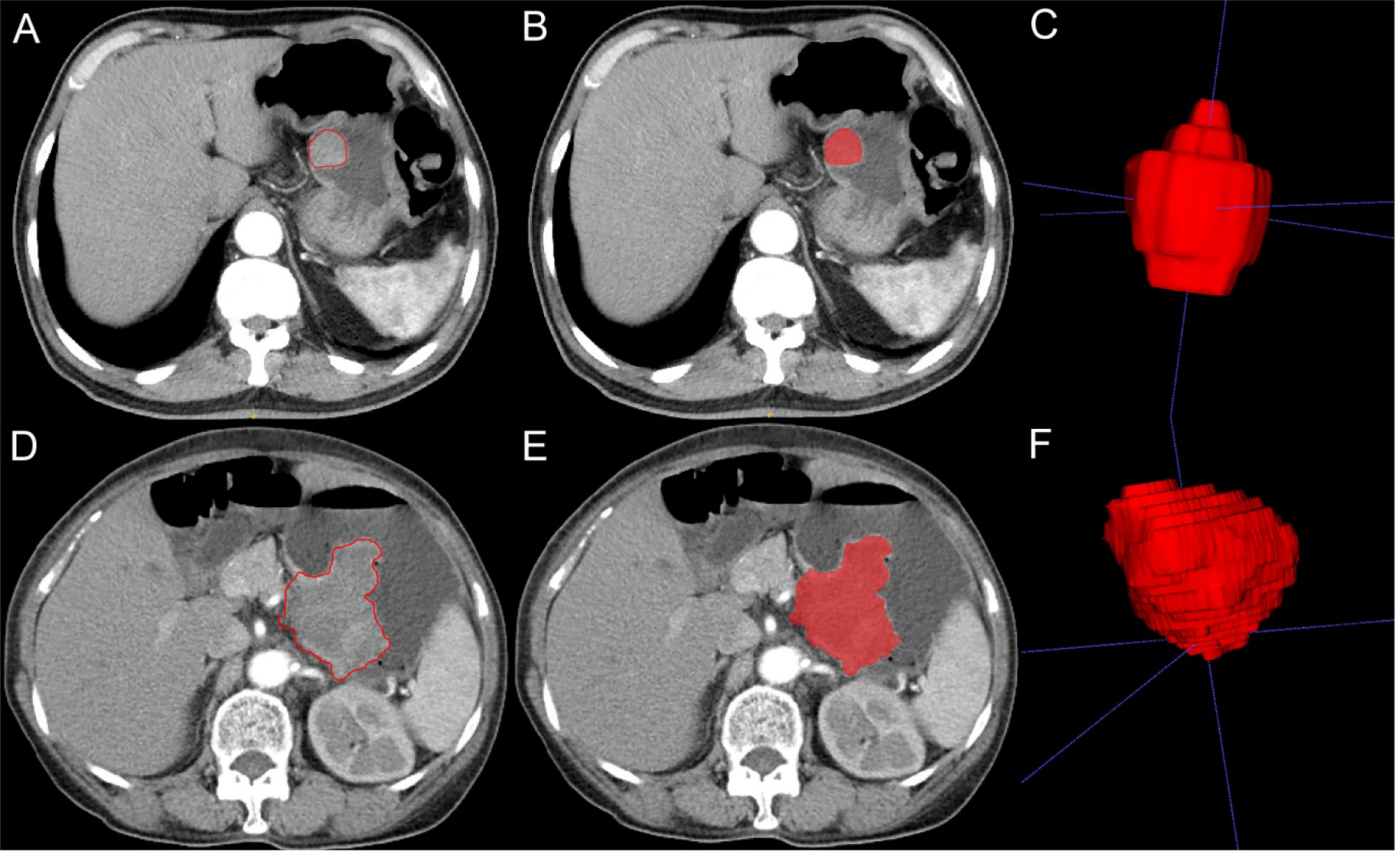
Imaging segmentation of gastrointestinal stromal tumors (GIST) on computed tomography (CT) imaging. (A) Two-dimensional (2D) CT arterial phase image of potential malignant GIST. The tumor is quasicircular and uniformly enhanced. The red outline is the boundary drawn by radiologists to show the tumor. (B) 2D segmentation of the tumor. (C) 3D segmentation of the tumor lesion. (D) 2D CT arterial phase image of malignant GIST with irregular shape and uneven internal enhancement of the tumor. The red outline is the boundary drawn by radiologists to show the tumor lesion. (E) 2D segmentation of the tumor. (F) 3D segmentation of the tumor.
